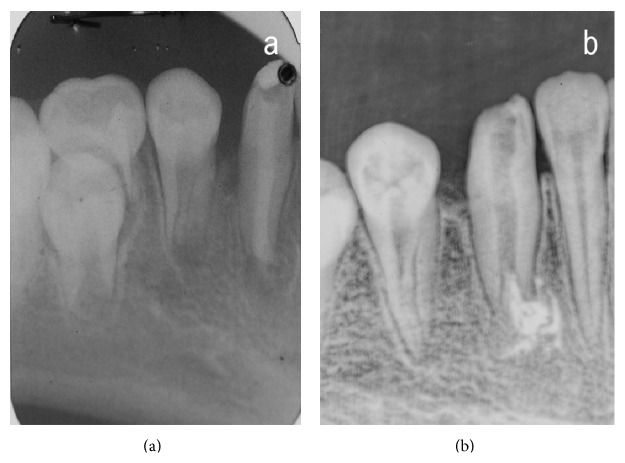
Lower periapical radiograph. (a) Monitoring phase (2007). (b) Treatment phase (May 2009).
